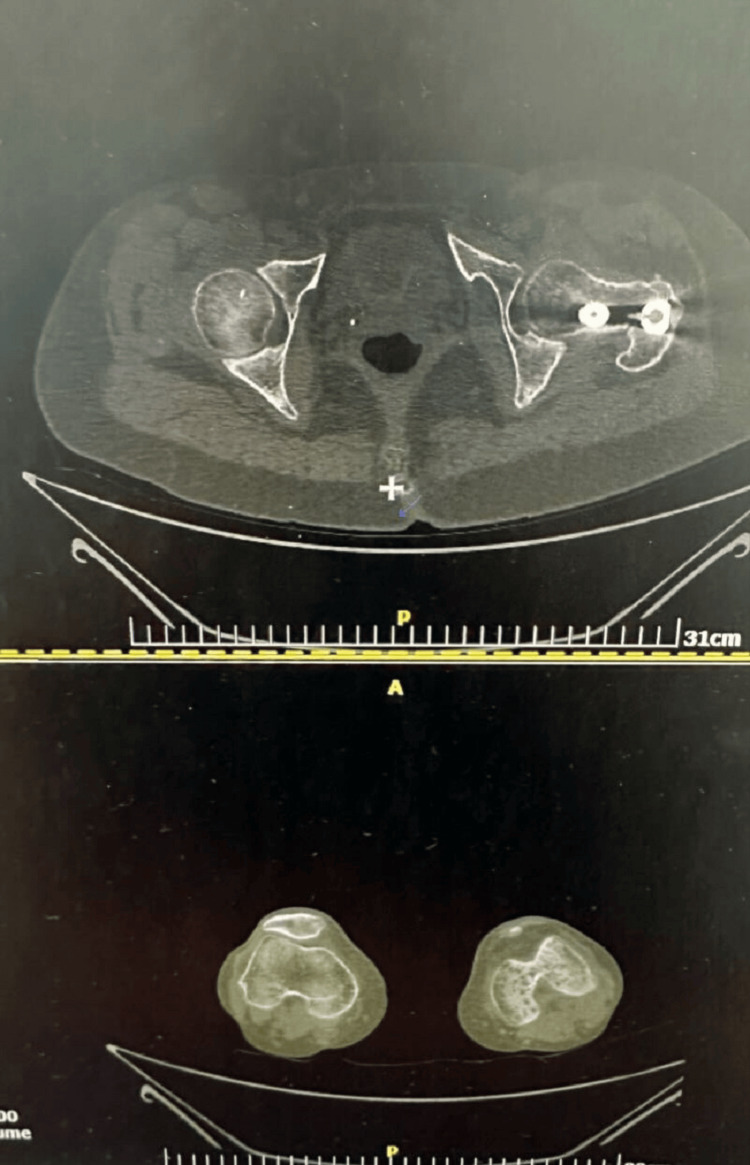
AV measurement Axial images from the postoperative CT scan used to calculate the femoral AV angle. AV, anteversion
